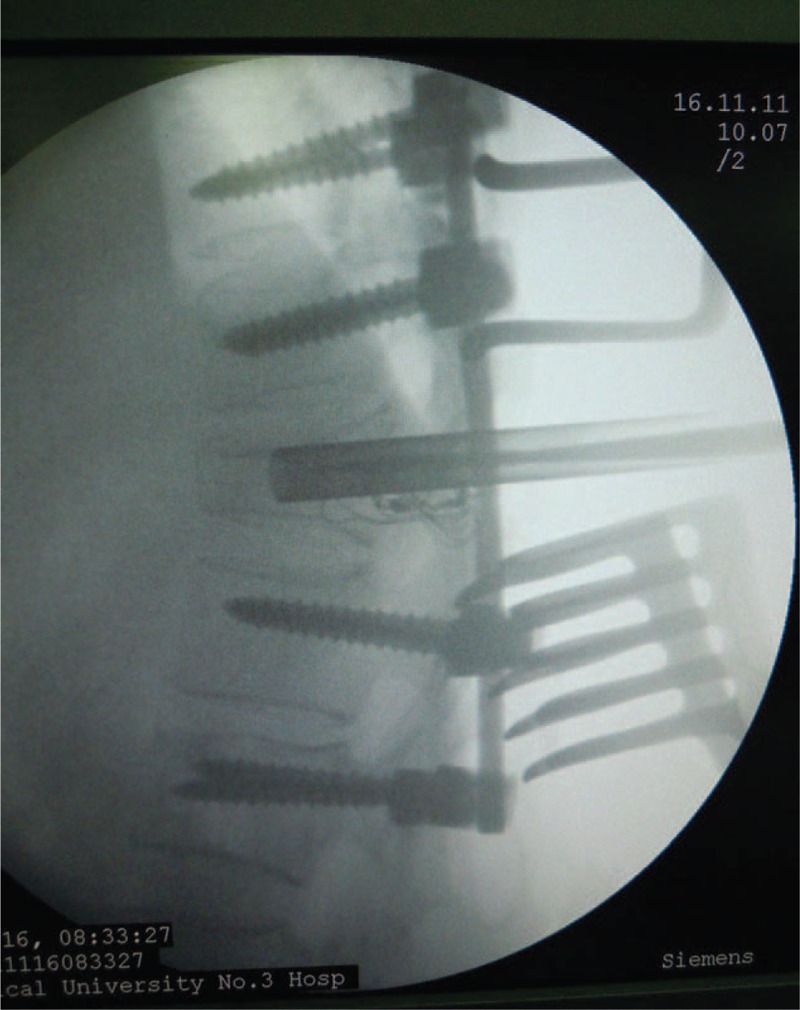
Intraoperative X-ray of the modified trephine being introduced into the vertebrae through the probe.
